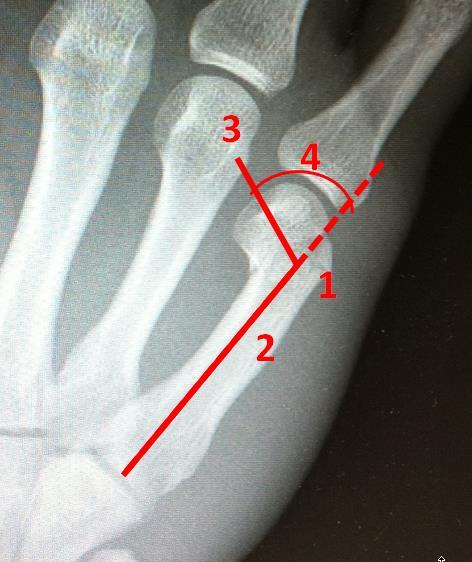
X-ray with fracture as used in the study.
